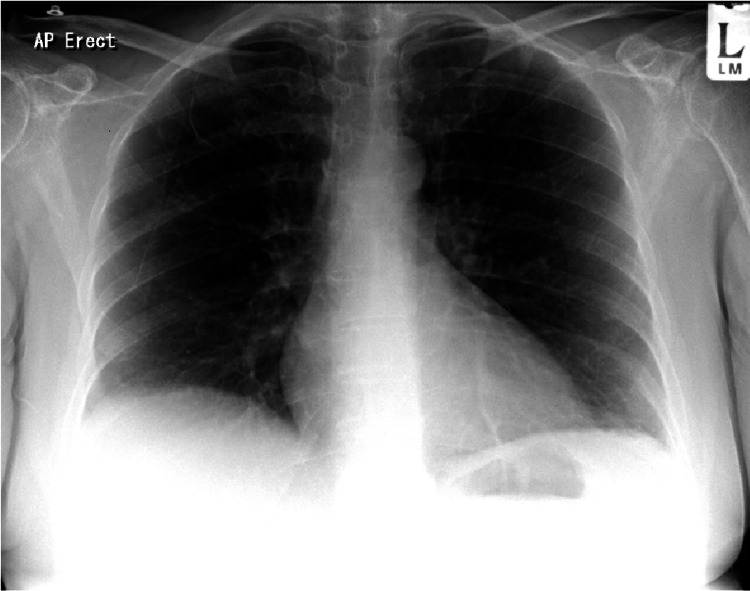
Initial normal chest X-ray
